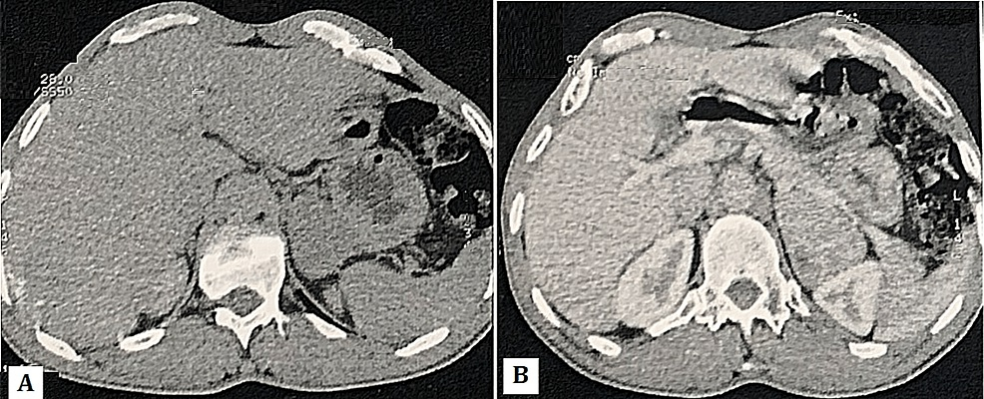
(A) Adrenal CT, axial section: left adrenal lesion, solid-necrotic with a spontaneous density of 40UH. (B) Axial image on portal phase (CT) scan showing a left adrenal mass, heterogeneously enhanced
